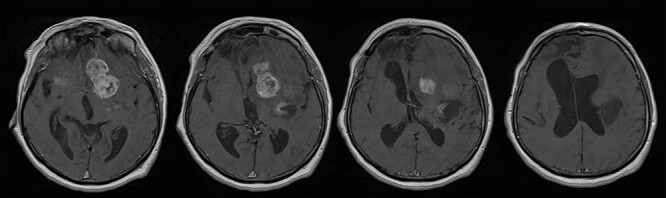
Brain MRI scans revealed an irregular expansive multicenter process located in the basal ganglia, frontobasal and in the area of the frontal operculum left and frontotemporal right with extensive vasogenic edema of the left frontal and parietal lobe and a compressive effect on the left lateral and III ventricle; after the administration of intravenous contrast, the process showed heterogeneous enhancement. Ventricular system was dilated with sign of transependymal edema.
